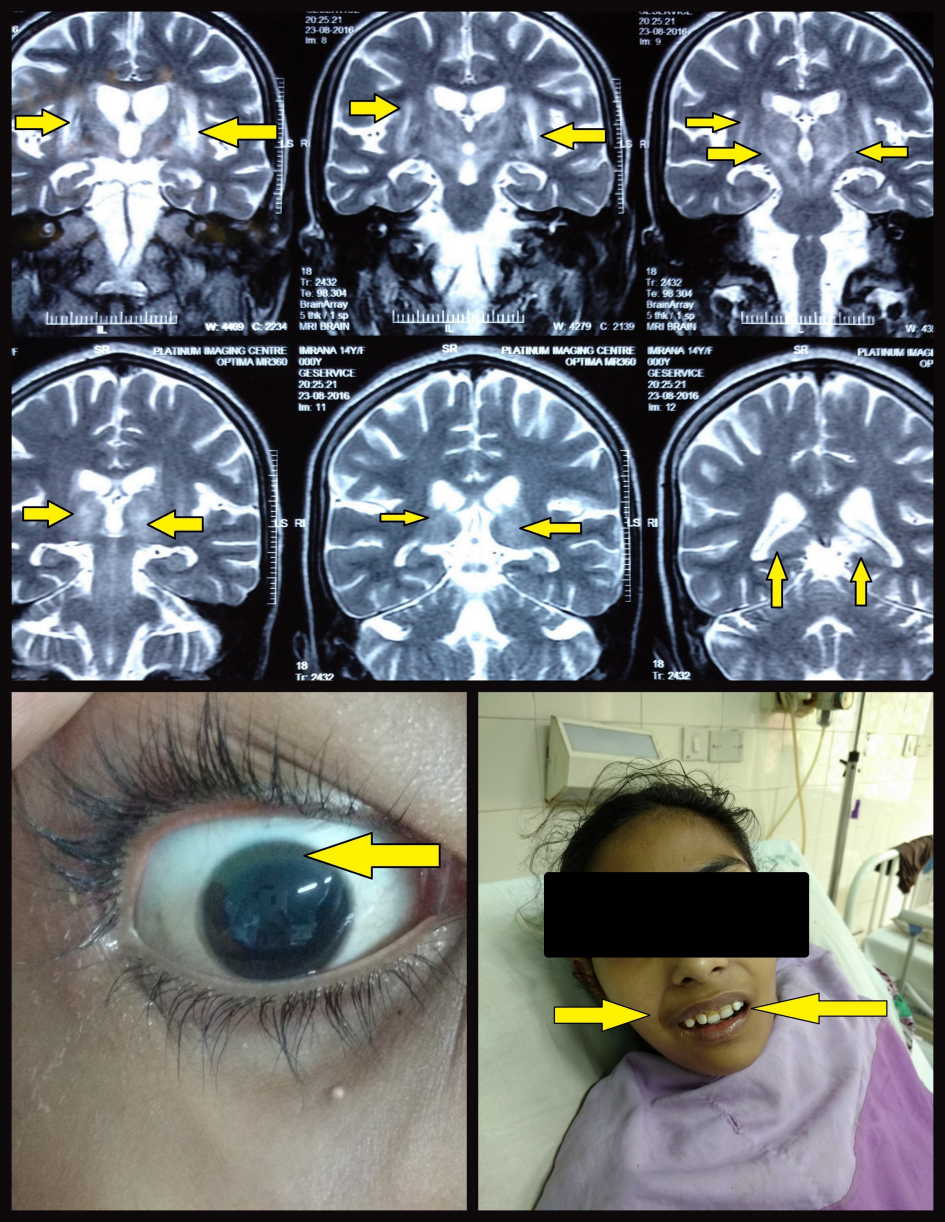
Figure Showing Hyperintensity of Basal Ganglia and Surrounding Brain Gray Matter on MRI, Kayser-Fleischer Rings, and Mask-Like Facies
